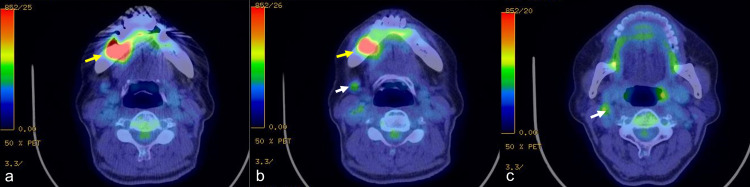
Positron emission tomography Positron emission tomography-CT showed uptake of 18F-2-fluoro-2-deoxy-D-glucose (FDG) in the mandibular gingiva with a maximum standardized uptake value (SUVmax) of 14.5 (yellow arrow) (a-b). Uptake of FDG of two lymph nodes of the neck had an SUVmax of 2.0-2.3 (white arrow) (b-c).
